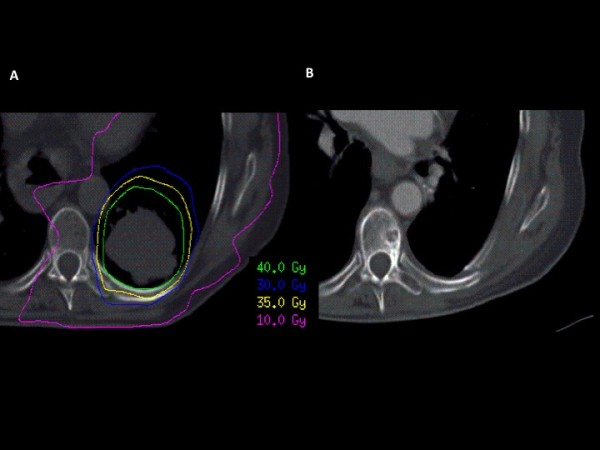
Radiation dose distribution in the transverse plane (A) and radiation dose distribution co-registered with CT images during the follow-up (B).
